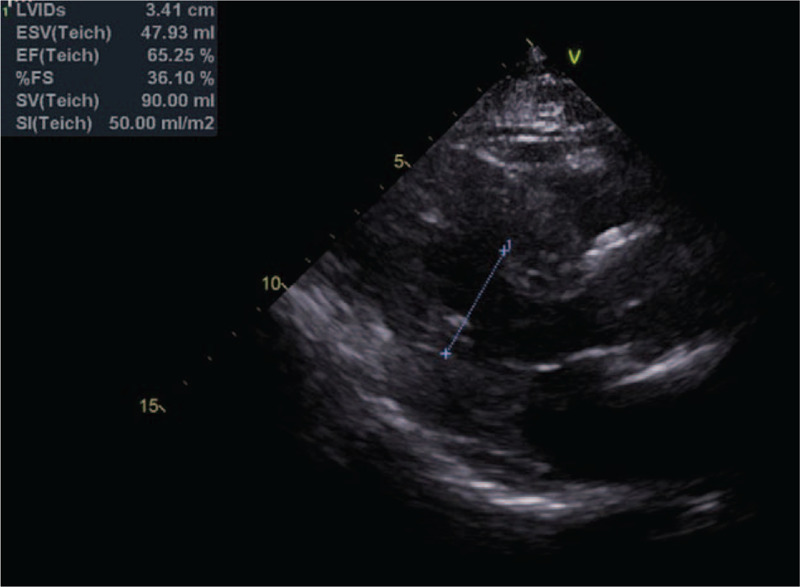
Preoperative transthoracic echocardiograph showing ischemic heart disease with preserved left ventricular ejection fraction (65%). EF = ejection fracture, ESV = endsystolic volume, FS = fractional shortening, LVID = Left ventricle internal diameter, SI = stroke index, SV = stroke volume.
